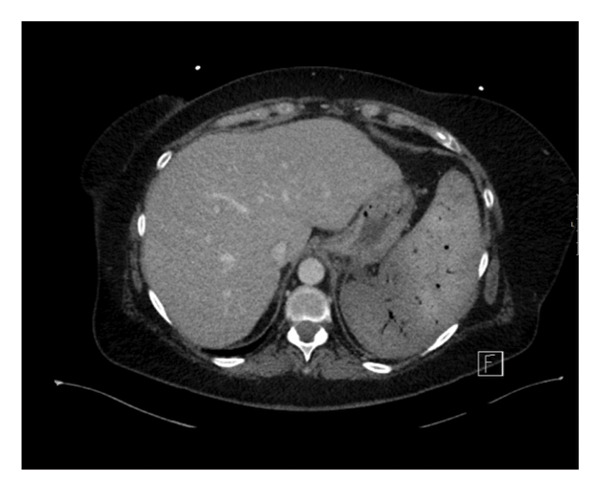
Complete infarction of the spleen on follow‐up CT (axial slice) of Case 2.
